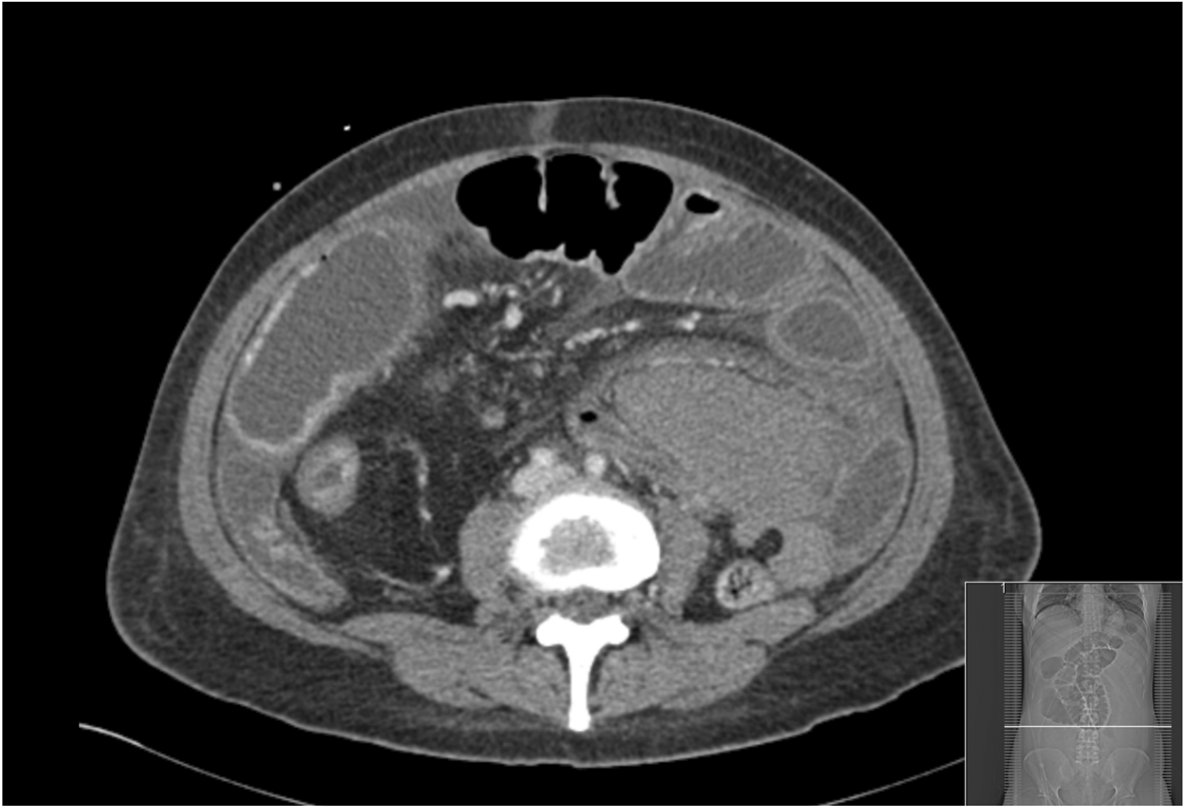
Computed tomography (CT) scan demonstrating small bowel obstruction and mesenteric haematoma taken at the same anatomical level as Figure1b.
